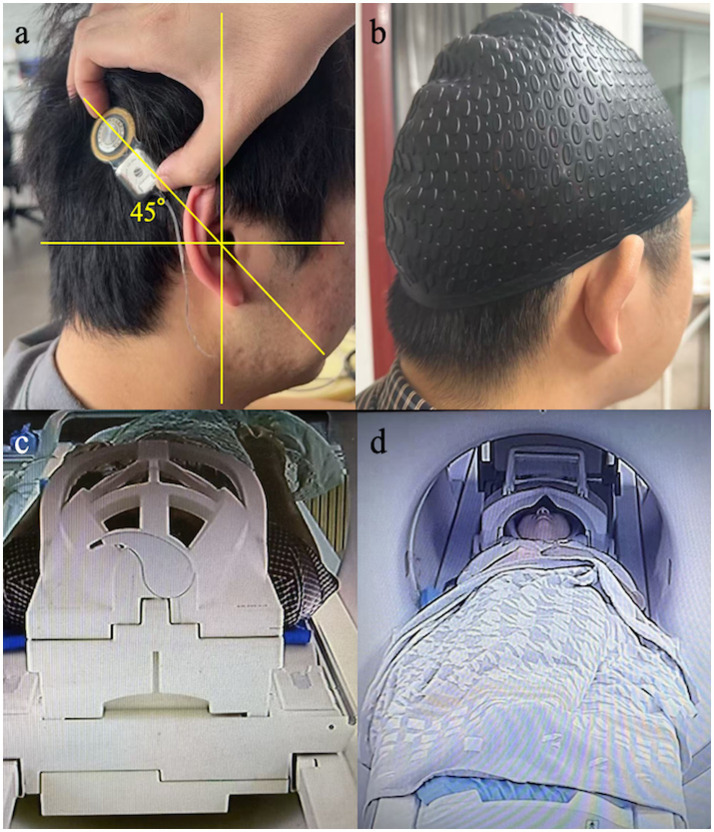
(a,b) The cochlear implant was fixed to the right temporal region of the head using a swim cap, and the implant position was marked on the surface of the cap (c,d) MRI scans were performed under 1.5 T and 3.0 T static magnetic field conditions.
